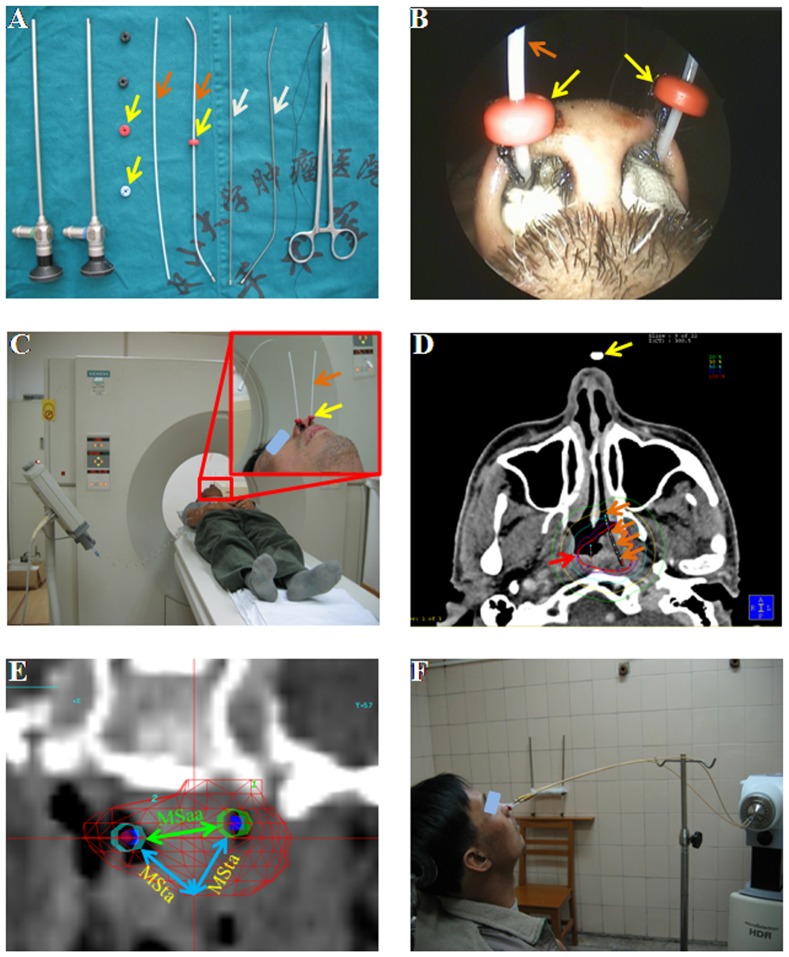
Endoscopic-guided IMBT boost administration process. (A) The instruments and applicators used in endoscopic-guided IMBT boost process. (B) The nasal outward view of applicators sewed to the nose wings. (C) CT scan of the tumor and applicators location. (D) CT images of tumor residue, applicators and isodose line. The red arrow indicated the 100% isodose curve covering the whole GTV. (E) The 3-dimensional reconstruction image of two applicators (outlined by hepta-prism) inserting into the nasopharyngeal residue (outlined by red fine grid lines) in coronal section. MSta was referred to the maximum spacing from the tumor margin to the nearest applicator (blue arrows), and MSaa was referred to minimum distance between two applicators (green arrow). (F) A representative case that was delivering the IMBT boost. The crimson arrow denoted the applicator outline, and white arrow displayed the 6F sharp Obturator with 0 and 30 degree angle. The yellow arrow indicated the Radio Opaque Button.
